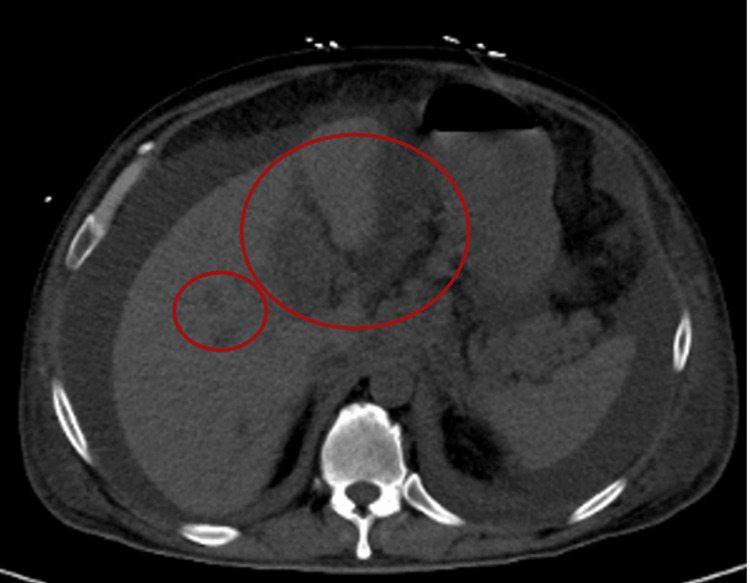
CT image showing a complex mass involving the liver and gallbladder (larger red circle) as well as additional hepatic lesions (smaller red circle), concerning for metastatic disease.
